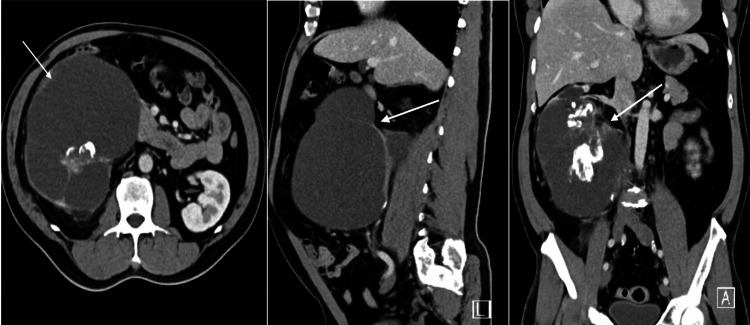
CT scan of abdomen and pelvis with contrast CT scan images showed a large right multilocular cystic mass (white arrows) in axial, sagittal, and coronal planes.
